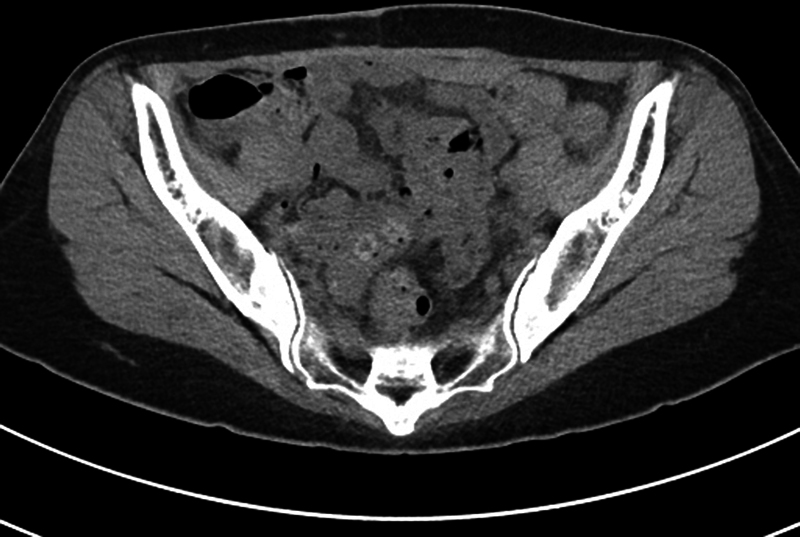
Axial contrast-enhanced CT image of case 2. Postoperative 3 months. CT, computerized tomography.
